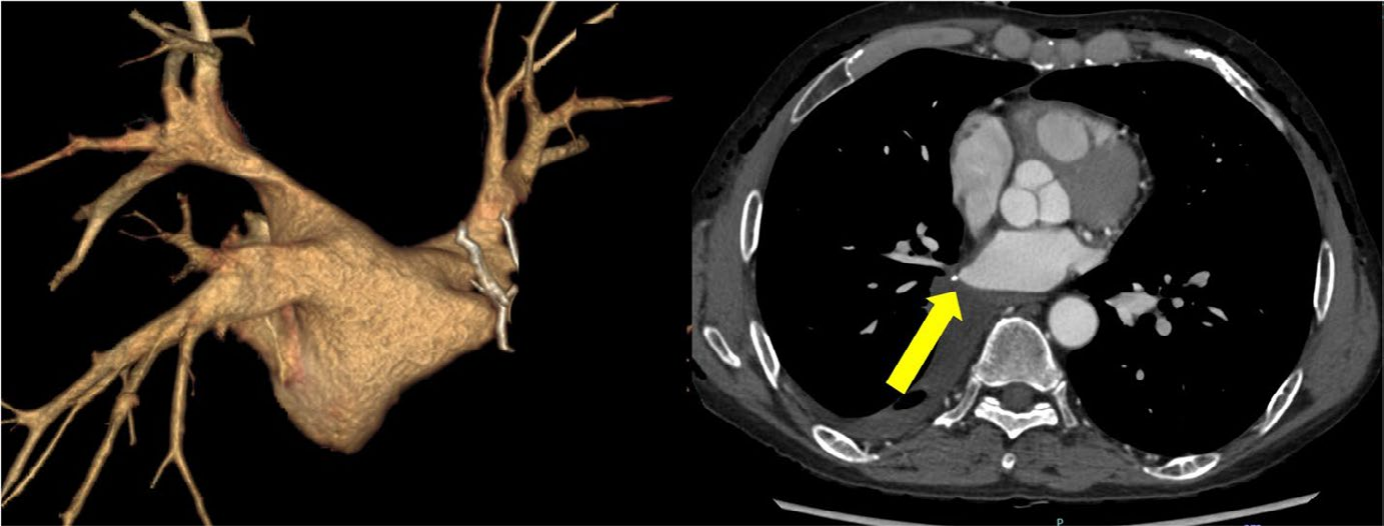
Case 2 had undergone right lower lobectomy, resulting in the right inferior pulmonary vein (PV) being transected. Contrast‐enhanced CT shows that the right inferior PV was the PV stump (yellow arrow).
